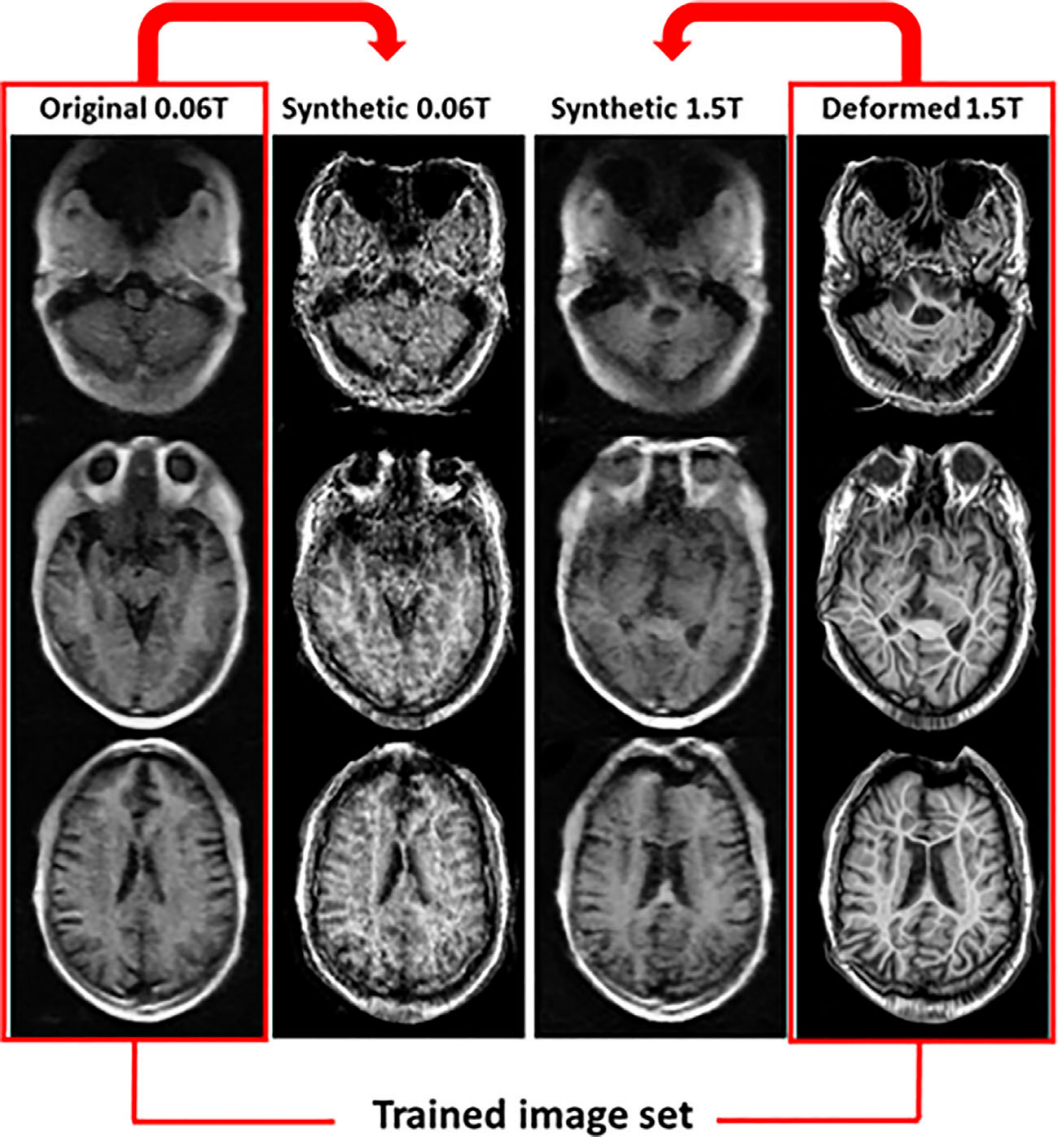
Synthetic MR images from original 0.06 Tesla and deformed 1.5 Tesla MR images in step 2 (cyclic-GAN model, method 2).
